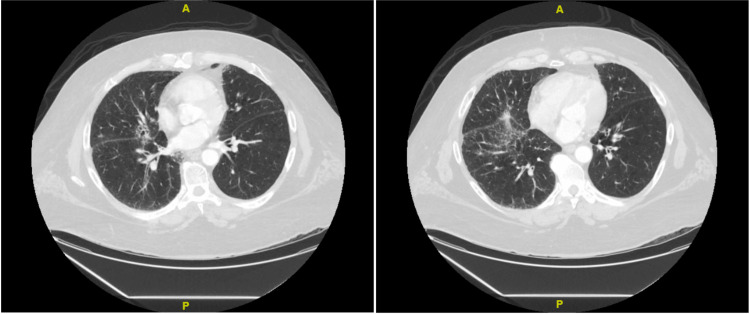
Chest CT The chest CT is suggestive of a diffuse and invasive type of adenocarcinoma with lymphangitic carcinomatosis.
